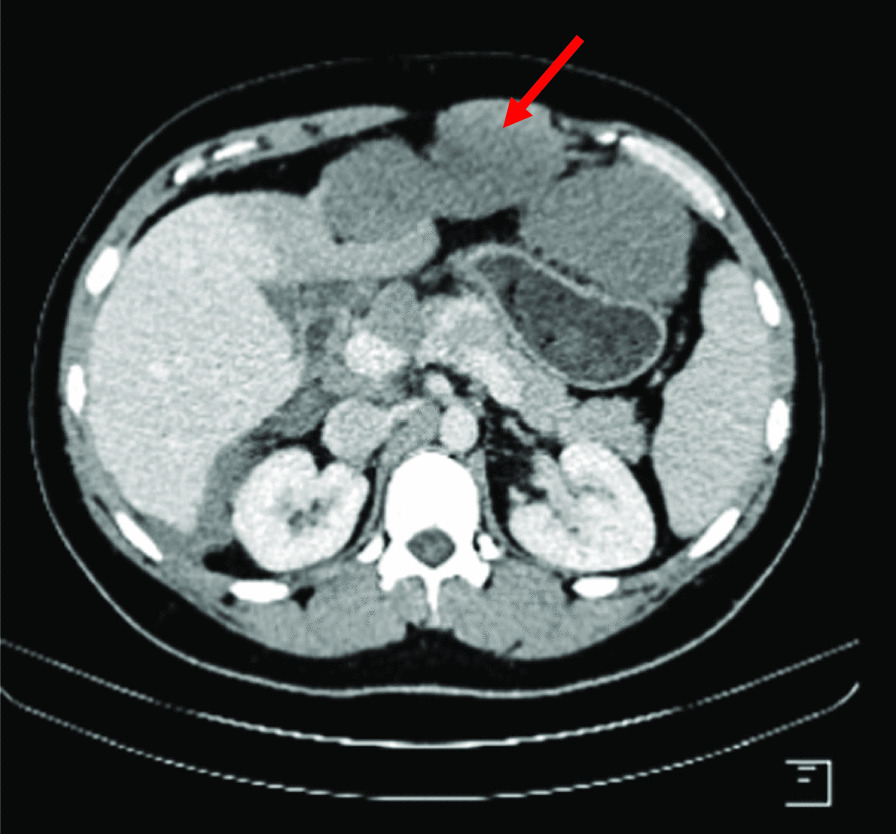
Case 3: axial section of abdominal CT scan with injection at venous time, showing invasion of the left rectus abdominis muscle by the tumor (red arrow)
